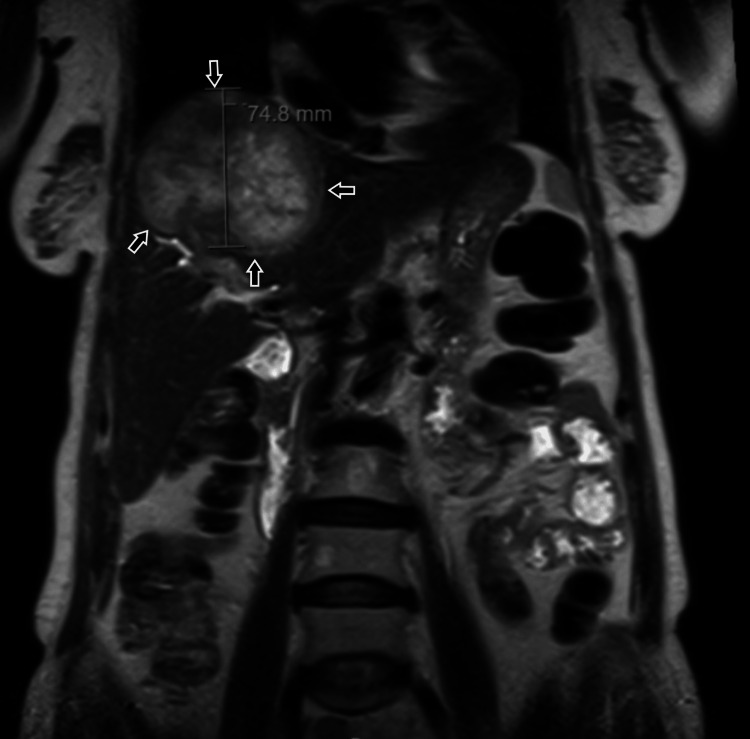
Abdominal and pelvic MRI with large hepatic nodule - coronal view MRI: magnetic resonance imaging
